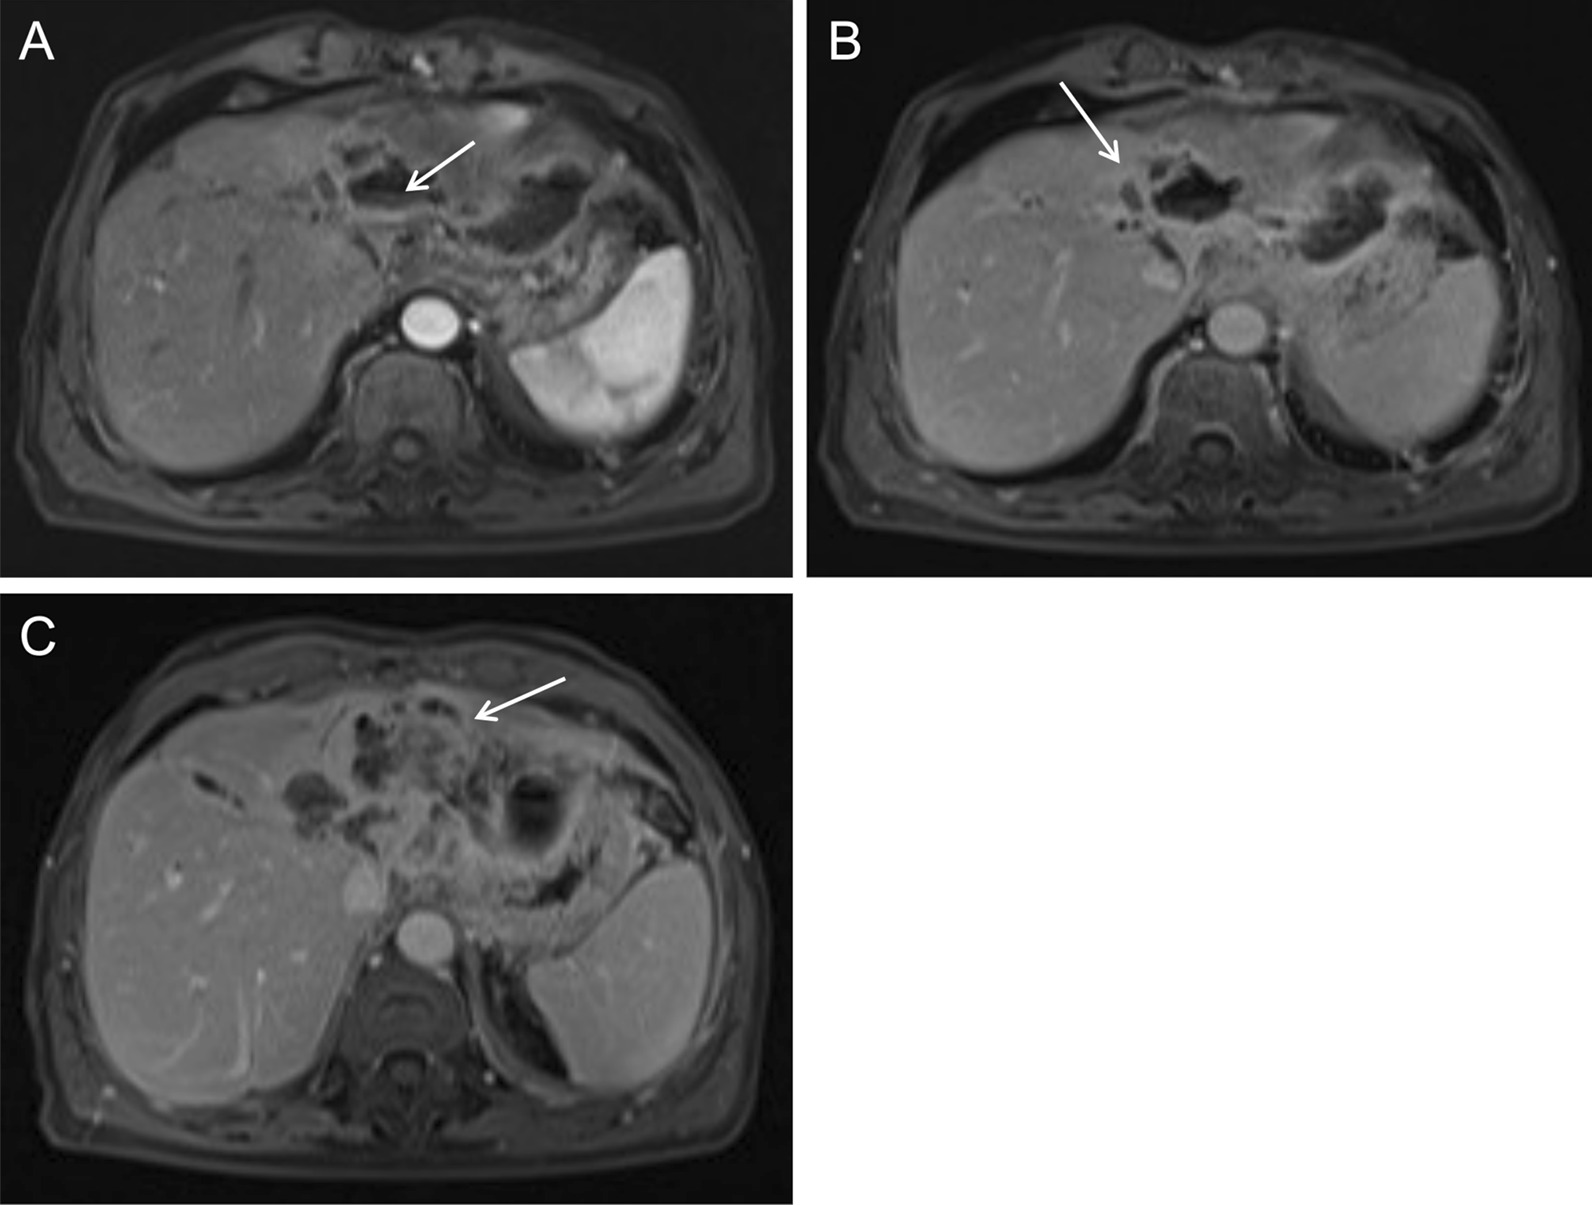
Enhanced MRI images of case 3
